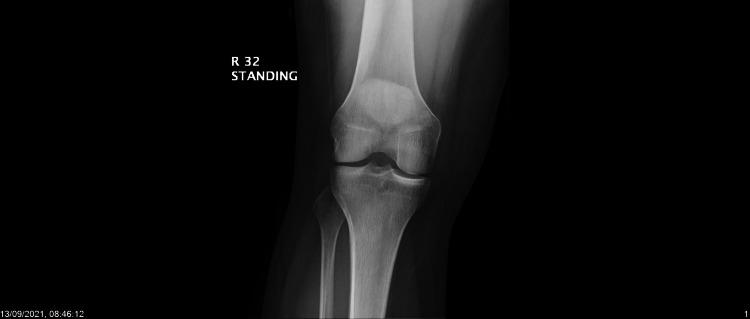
Anterior right knee plain radiograph
